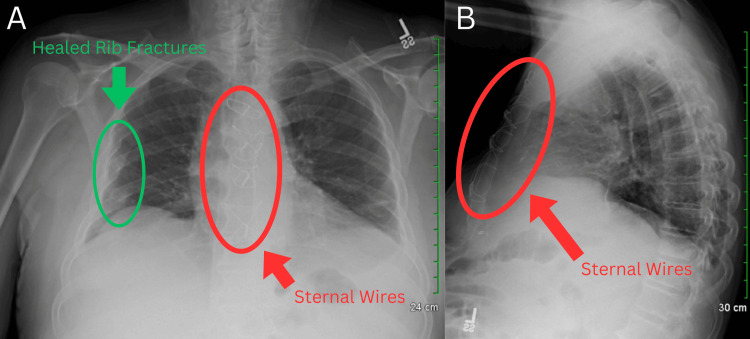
Anteroposterior (A) and lateral (B) chest X-rays showing a normal cardiac silhouette, presence of sternal wires (A-B), old healed right rib fractures (A), reduced lung volumes, and clear lungs.
